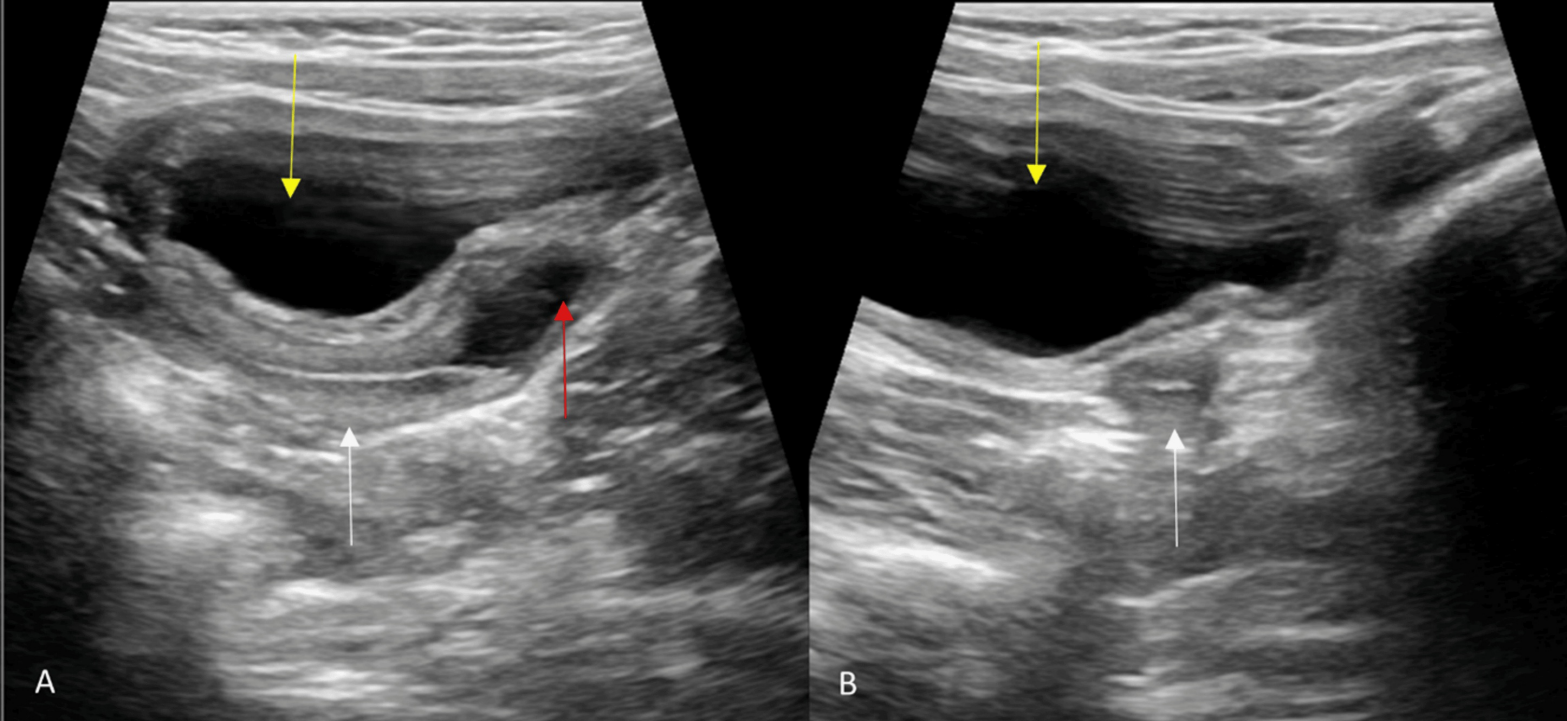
Pelvic ultrasonography images: A. Sagittal image shows the uterus (white arrow), mild fluid in the vagina (red arrow), and urinary bladder (yellow arrow); B. Axial image shows the uterus (white arrow) and urinary bladder (yellow arrow)
